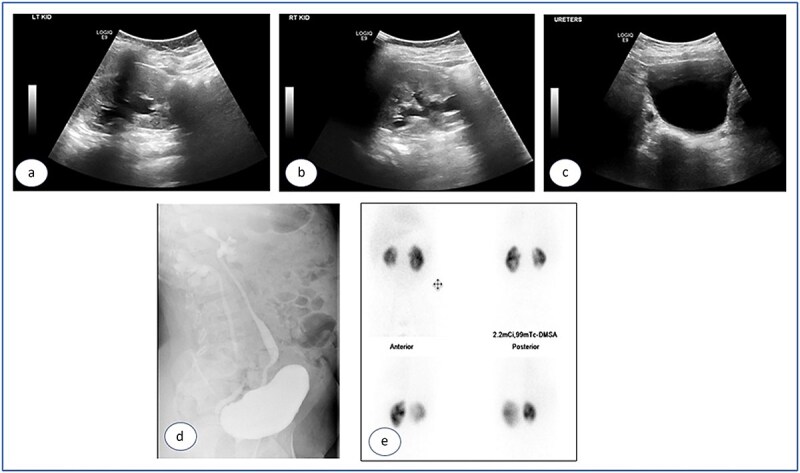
Ultrasound images taken 1 month after the removal of both double J stents, showing no hydronephrosis in either kidney—the left (a) and right (b)—and urinary bladder with Deflux in place (c).
